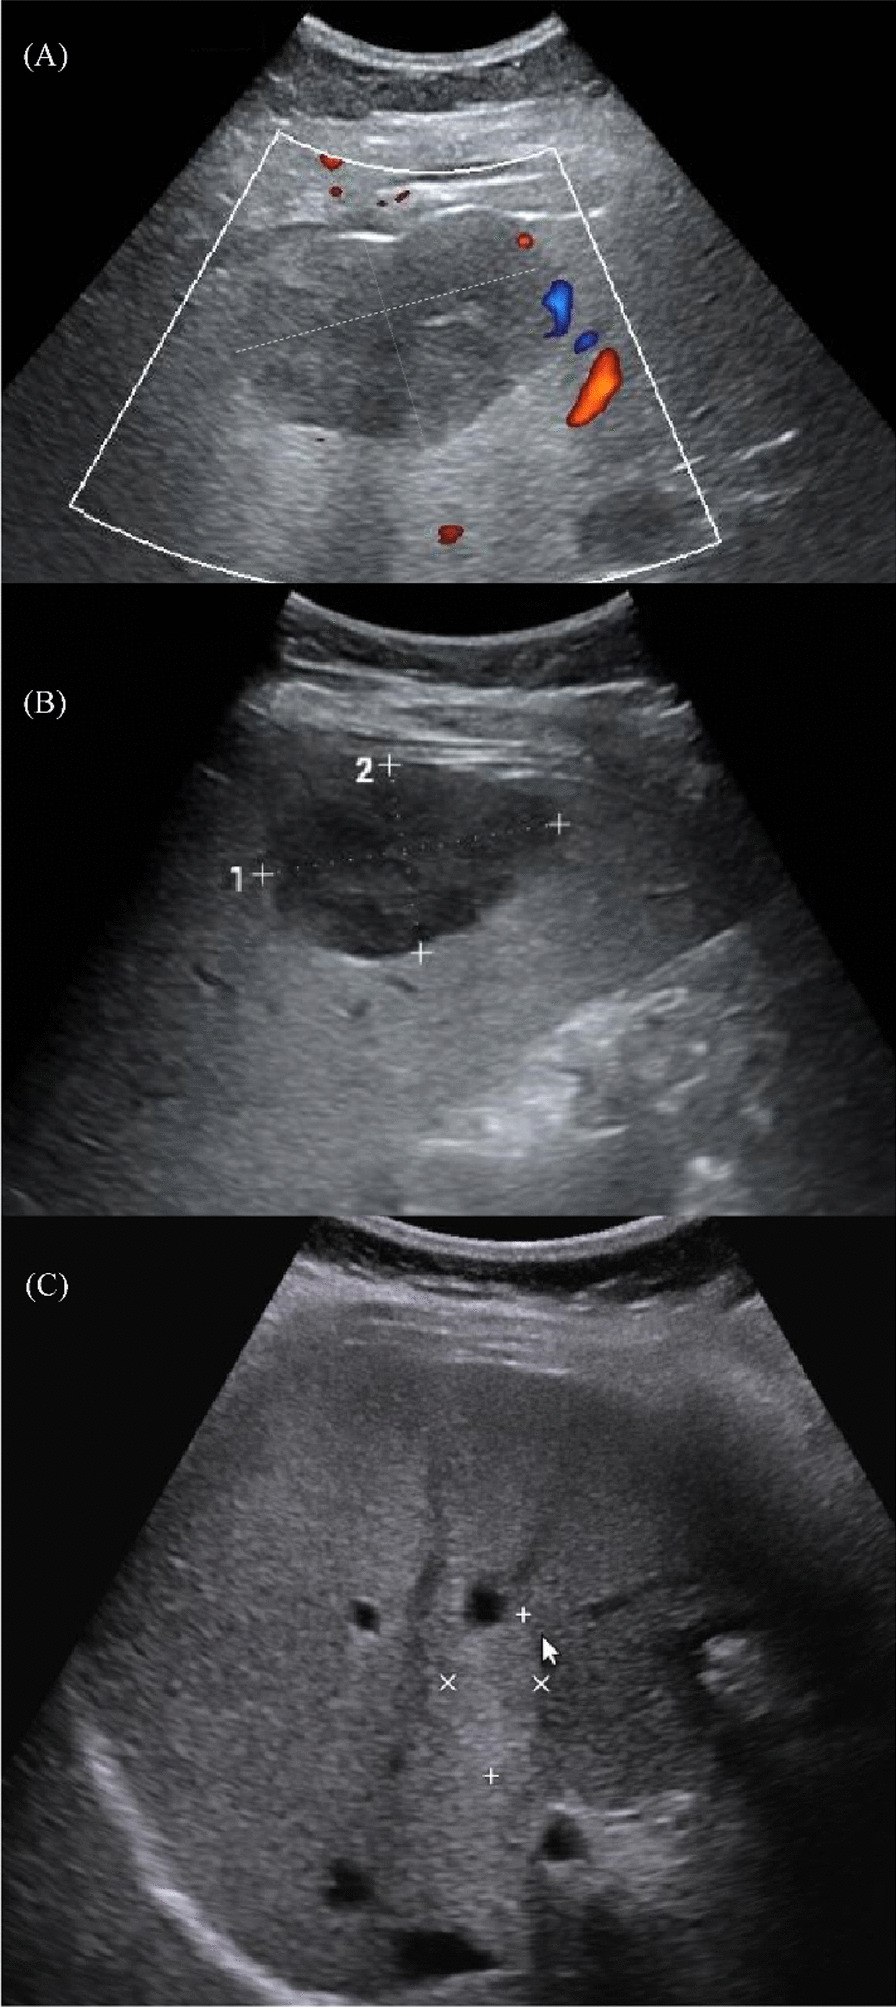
A 41-year-old female with a hepatic hemangioma of 6.0 cm in diameter. A Preoperative ultrasound (size: 6.0 cm × 3.7 cm). B Ultrasound after 6 months of the first percutaneous sclerotherapy. (size: 5.5 cm × 3.5 cm). C Ultrasound after 24 months of the second percutaneous sclerotherapy. (size: 2.7 cm × 1.6 cm)
